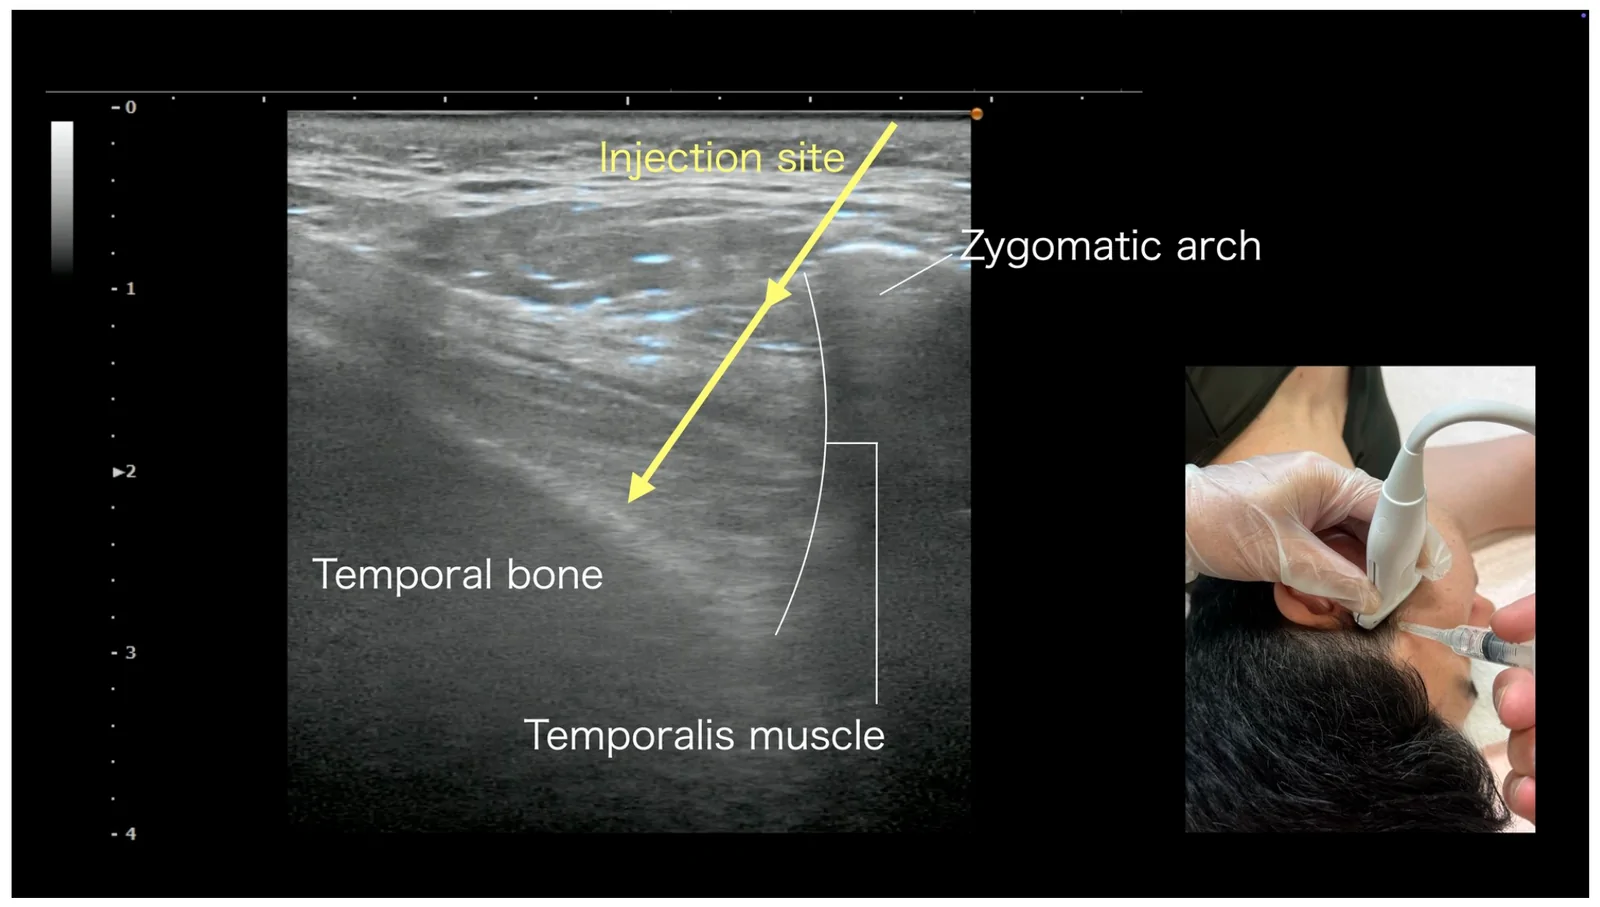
Temporalis US-FHR (POINT 2). Ultrasound image: Temporalis muscle, zygomatic arch, and temporal bone are identified. The yellow arrow (injection site) indicates the plane between the epimysial layers within the temporalis. With a 27 G 38 mm needle, 1–2 mL of saline is injected via the out-of-plane approach. The inset (bottom right) shows the patient position and probe placement.
